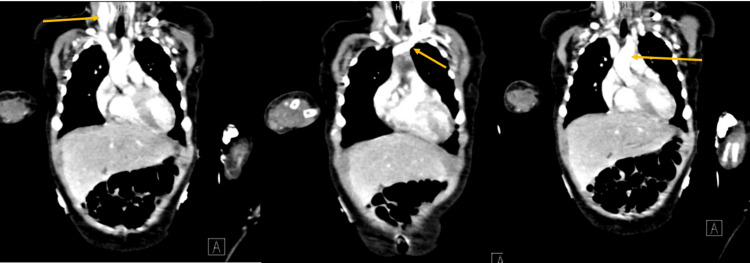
CT angiography showing multiple aneurysms
